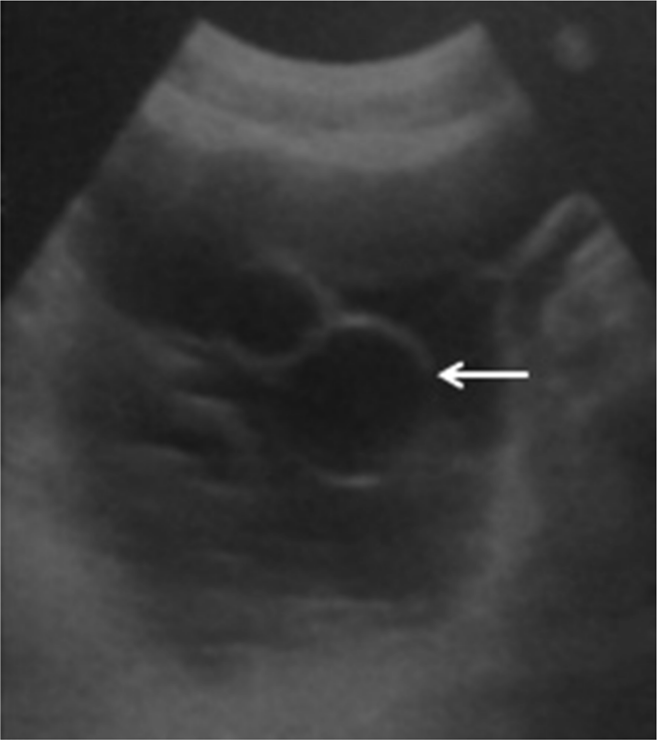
Abdominal ultrasonography shows a large amount of fluid and cysts into the peritoneal cavity (Arrow).
